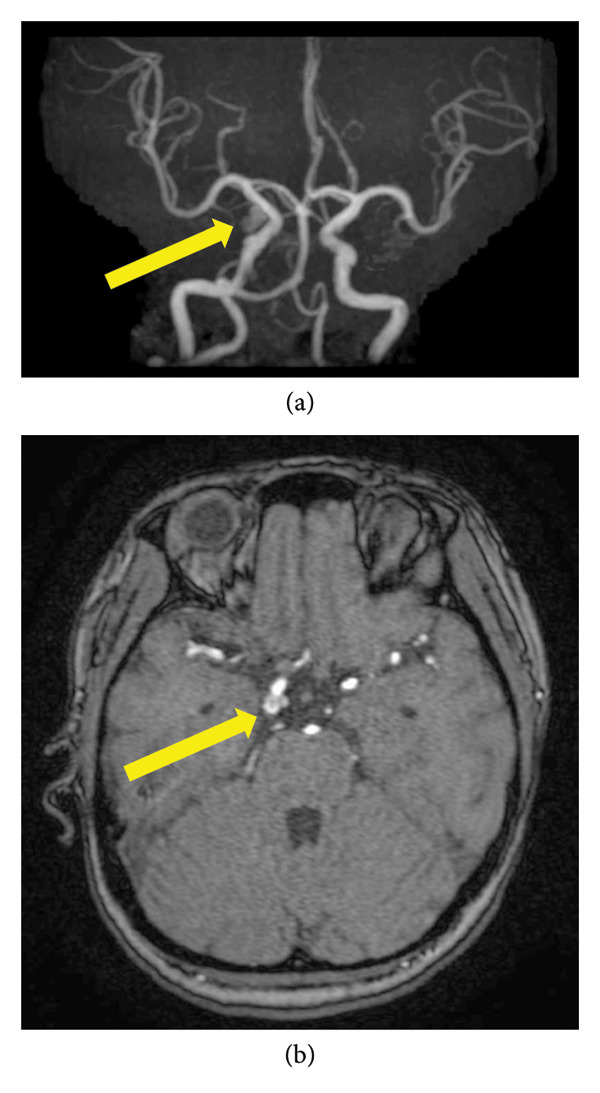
Preoperative brain magnetic resonance imaging findings. An 8‐mm cerebral aneurysm can be seen at the right internal carotid artery–posterior communicating artery bifurcation. (a, b) Axial section (a) and magnetic resonance angiography (b) showing the aneurysm (yellow arrows).
